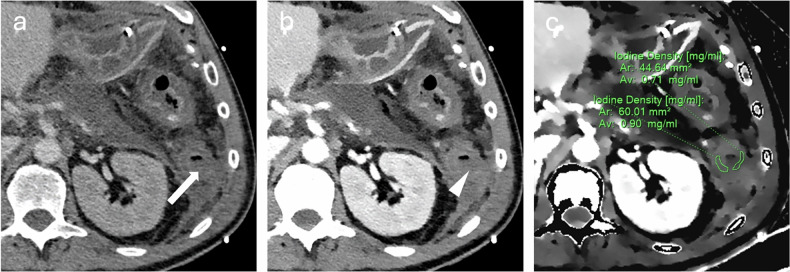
A 65-year-old man admitted to the intensive care unit for severe biliary acute pancreatitis (computed tomography (CT) severity index = 10), complicated by mesenteric ischemia requiring two ileocolic resections. The patient presented with hemodynamic and septic shock associated with abdominal pain. Abdominal dual-energy CT (DECT) was performed for suspected non-occlusive mesenteric ischemia (NOMI). a Axial 70-keV virtual monoenergetic imaging (VMI) shows the absence of enhancement of the bowel wall at the splenic flexure (arrow). b Axial 50-keV VMI confirms the lack of wall enhancement (arrowhead). c The iodine density map generated with DECT demonstrates residual iodine uptake within the bowel wall at the ROI locations (0.71 and 0.90 mgI/mL). Surgical exploration within 48 h led to resection of the affected bowel, and pathological examination showed an isolated mucosal necrosis
